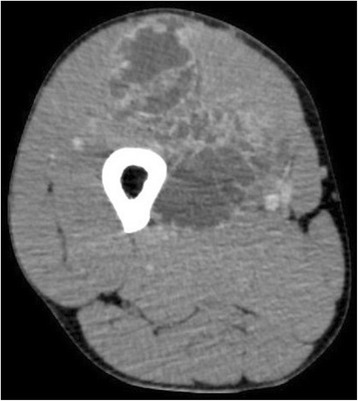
Computed tomography reveals a tumor without calcification or femur infiltration
